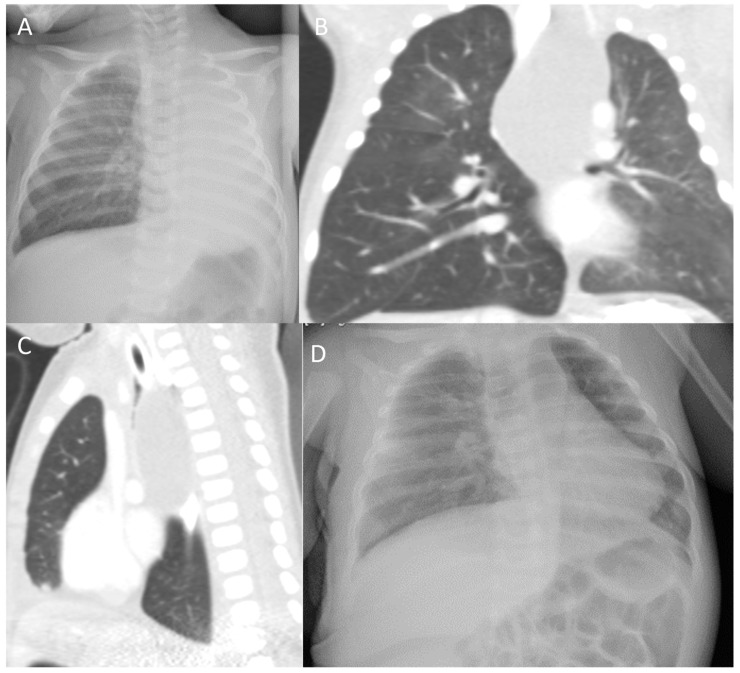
Chest X-ray (CXR) and chest computed tomography (CT) findings in case 1: (A) CXR of the patient on admission shows right lung hyperlucency, complete collapse on the left side, and marked left-sided mediastinal shift. (B,C) Coronal and sagittal windows, lung view; CT images of the chest reveal a well-defined cystic lesion in the posterior mediastinum at the level of the left apical lobe extending downward to the level below the carina with fluid density. The mass lesion attaches to the adjacent vessels and compresses the aortic arch, the descending aorta to the left side, and the inferior vena cava to the right side. The lesion shifts the esophagus to the right side with no obvious communication. (D) CXR of the patient at 1-month postoperative follow-up showing a well-aerated lung.
